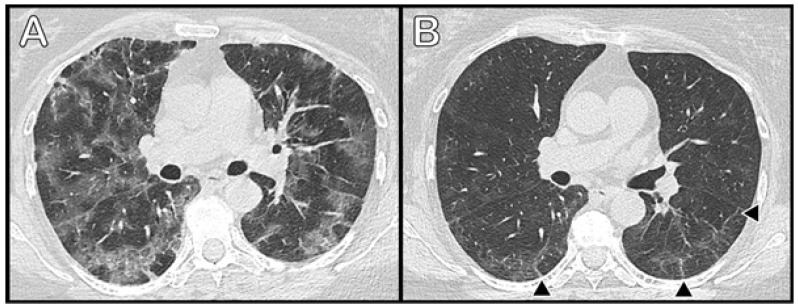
Axial thin-section chest CT images of a 60-year-old woman with confirmed COVID-19 pneumonia. (A) CT scan 10 days after the onset of symptoms at the peak stage of the disease. Multiple coalescing ground-glass opacities are seen in both lungs, with thickening of the interlobular septa in the lung periphery. (B) Follow up scan after 2 months. A decrease in density and size of ground-glass opacities can be seen, with persisting thickening of the interlobular septa and some parenchymal bands (black arrowheads) in peripheral parts of lungs.
